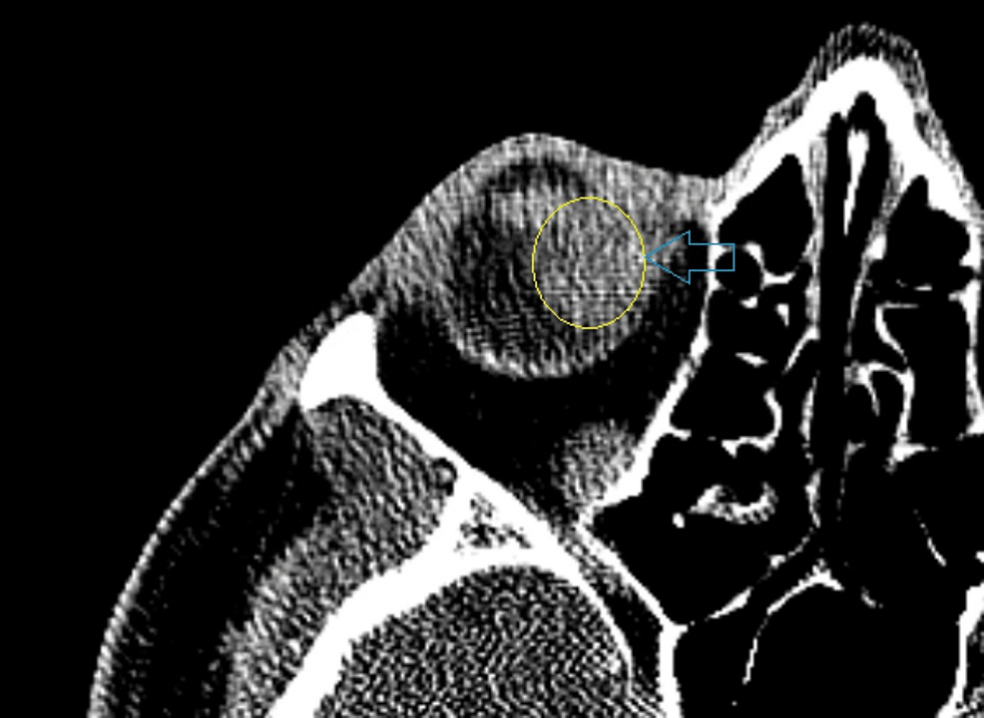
Computed tomography orbit, axial cut of uveal melanoma in the right (R) eye.
